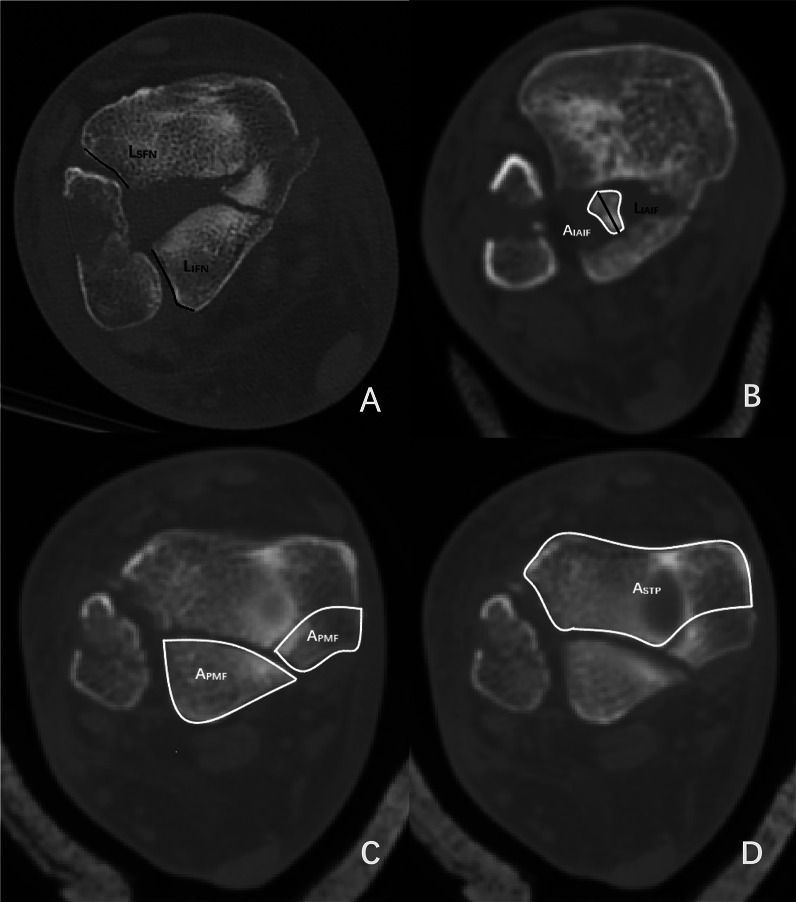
Example axial CT cross-sectional measurements. A Measured as the length of involved fibular notch (*L*_IFN_, length of involved fibular notch) and the stable fibular notch (*L*_SFN_, length of stable fibular notch). B Measured as the length and area of IAIF (*L*_IAIF_, length of IAIF, black; *A*_IAIF_, area of IAIF, white). C Measured as the area of posterior malleolar fragment (*A*_PMF_, area of posterior malleolar fragment). D Measured as the area of stable tibial plafond (*A*_STP_, area of stable tibial plafond)
